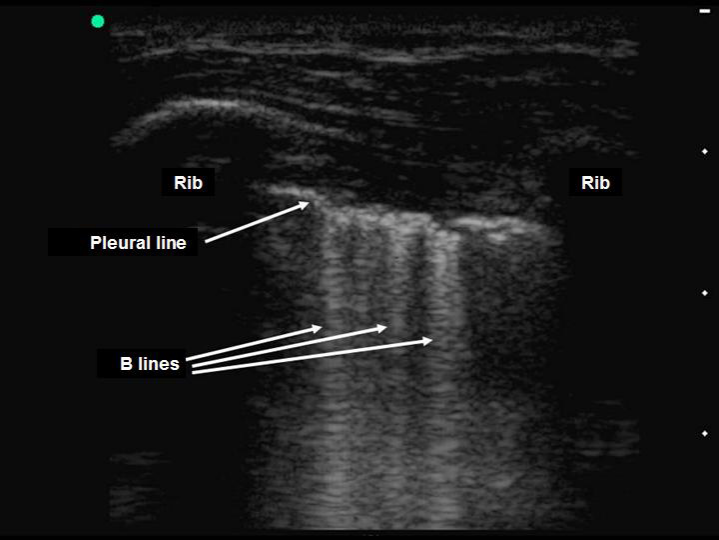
Detection of B-lines on pleural ultrasonography in two-dimensional mode. The presence of vertical linear artefacts arising from the pleural line (B-lines or comet-tail artefacts) rules out pneumothorax in this patient with interstitial syndrome. Reproduced with permission (ACCP - Chest).
